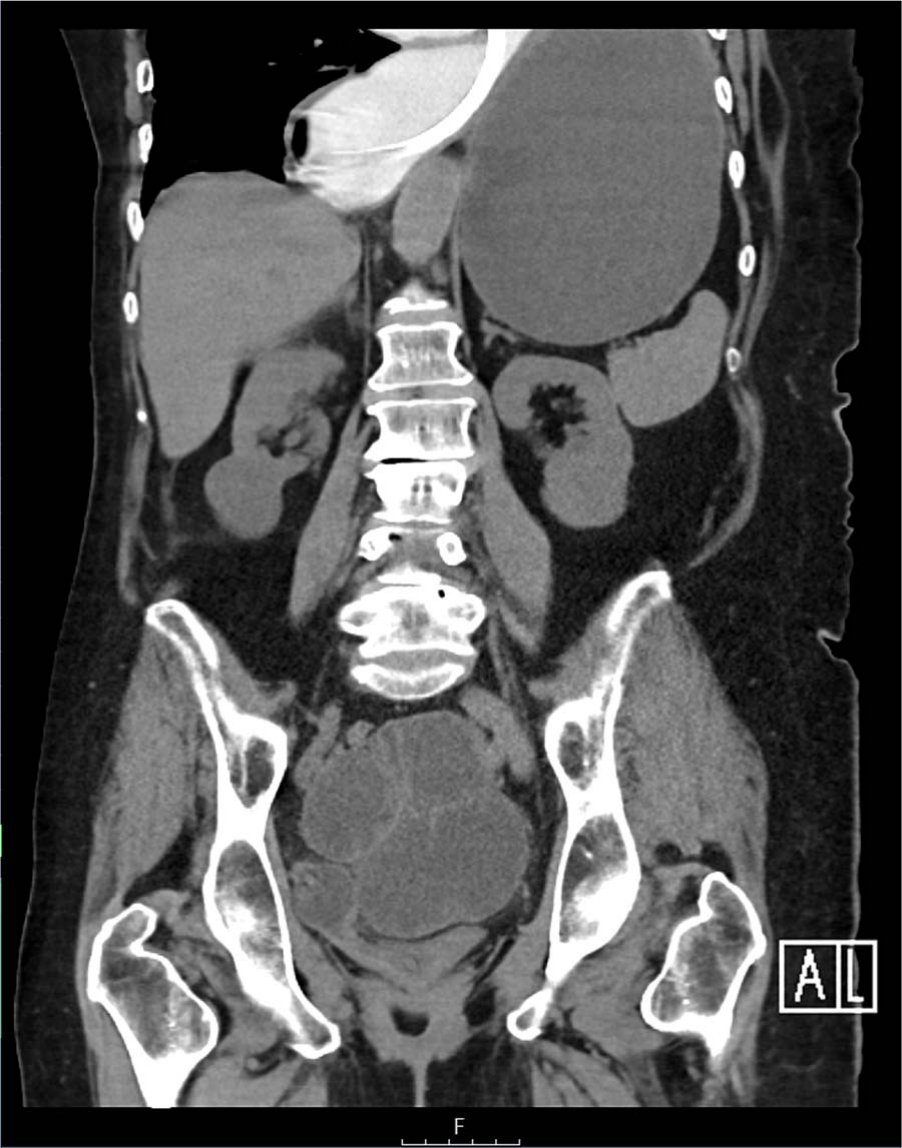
CT coronal view—NGT stuck in distal esophagus.
